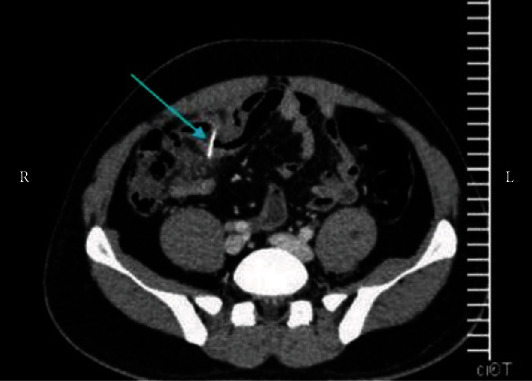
CT scan of case number 2 showing foreign body in the ileum (arrow).
